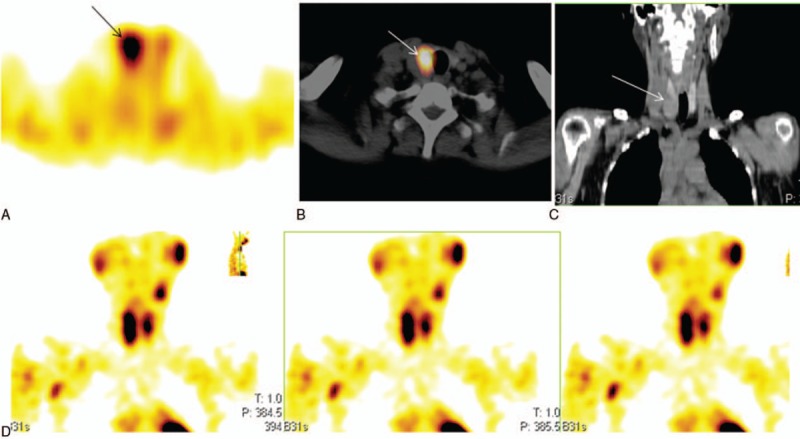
Dual-phase ^99m^Tc-MIBI SPECT/CT showed intense uptake in the right inferior parathyroid. (A) Transaxial image, (B) fusion image, (C) coronal CT, (D) coronal image. MIBI = methoxyisobutylisonitrile, SPECT/CT = hybrid single photon emission computed tomography/x-ray computed tomography.
